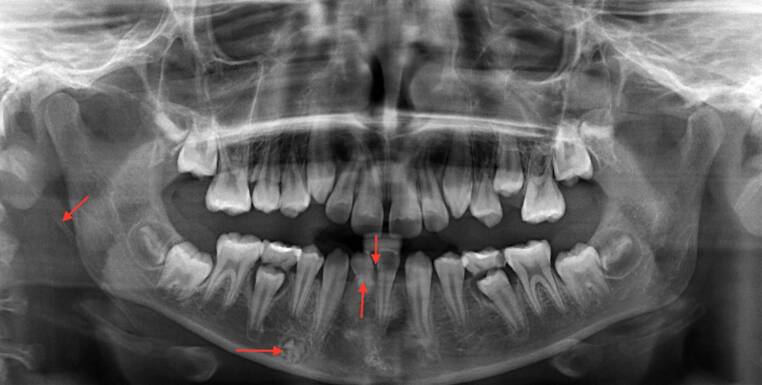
Orthopantomogram (OPT) of an 11-year-old boy with various incidental findings (*arrows*: reduced tooth number, dilaceration at tooth 42, suspected odontomas region 44 and calcified right-sided stylohyoid ligament) Orthopantomogramm (OPG) eines 11-jährigen Patienten mit diversen Nebenbefunden (*Pfeile*: Zahnunterzahl, Dilazeration am Zahn 42, Verdacht auf Odontome Regio 44 und kalzifiziertes Ligamentum stylohoideum rechts)
